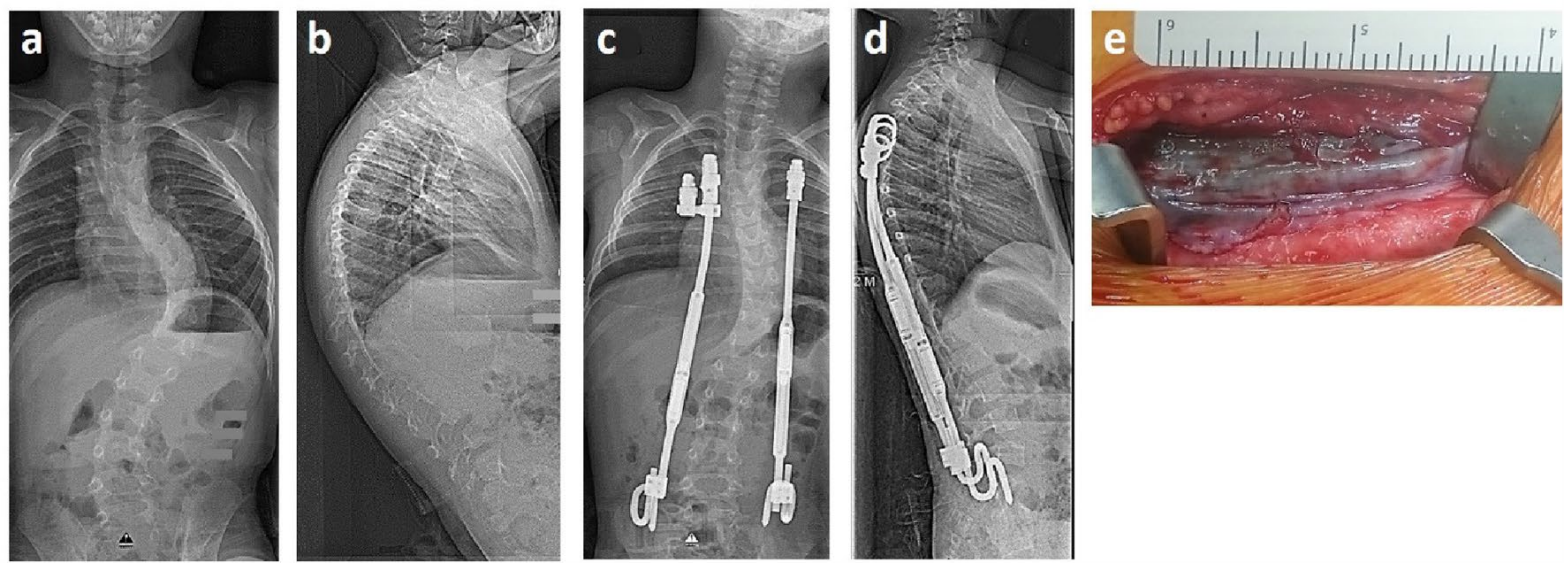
Bilateral MCGR system for spinal deformity correction in children. Anterior–posterior (a,c) and lateral radiographs (b,d) of a 6-year old boy with neuromuscular scoliosis. The main scoliotic curve was corrected from 71° (a) to 32° (c) with additional improvement of the sagittal profile (b,d). Intraoperatively, discoloration of the adjacent soft tissue was found (e).
